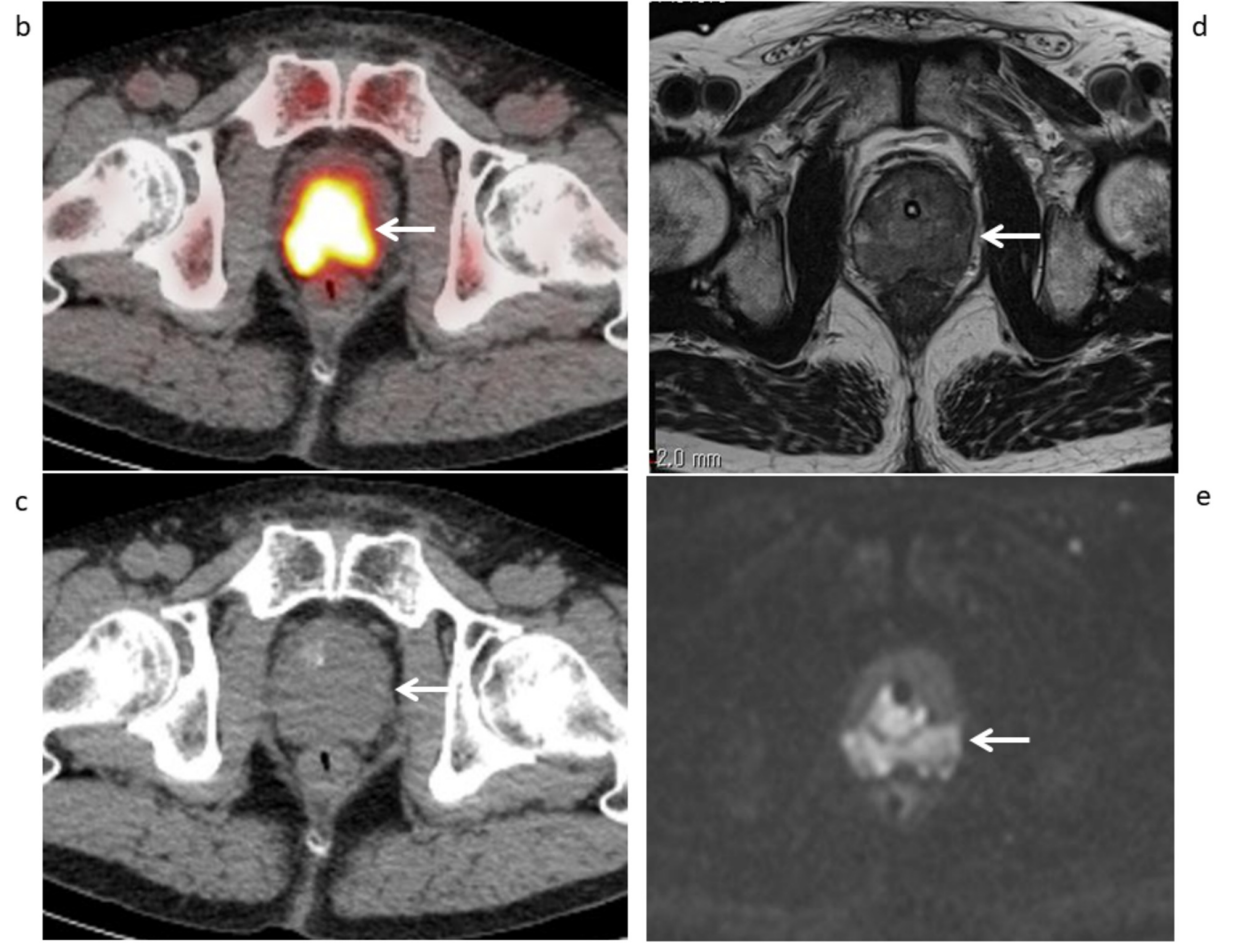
Axial 11C-choline PET/CT and pelvic MRI (Case 1) (b) Axial ^11^C-choline positron emission tomography/computed tomography (PET/CT) and (c) CT part show strong ^11^C-choline uptake (SUVmax 8.27) in the prostate gland (arrows), with a score of 5 for diagnosing local recurrence in the prostate gland. (d) Axial T2-weighted MR image shows hypointense area measuring 35×39 mm in the bilateral prostate gland with restricted diffusion on (e) DWI (arrows), with a score of 5 for diagnosing local recurrence in the prostate gland.
